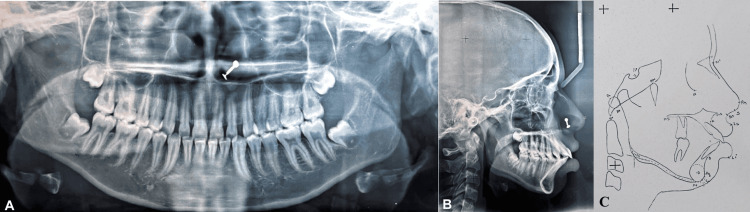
(A) Orthopantomogram. (B) Lateral cephalogram. (C) Tracing of lateral cephalogram.
